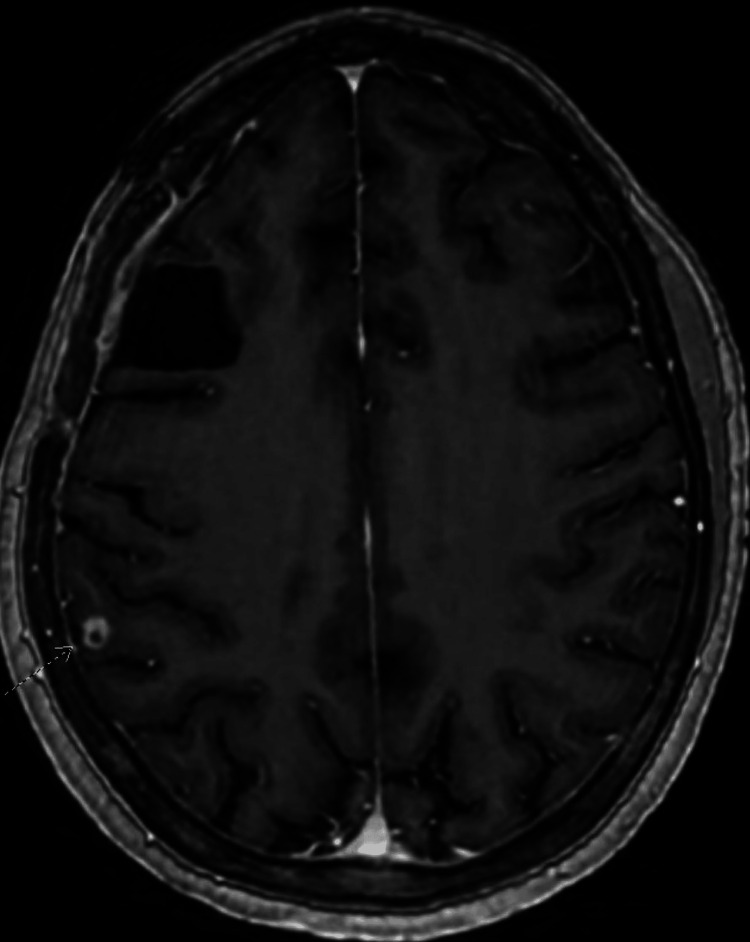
Follow-up brain MRI with and without contrast at four months after craniotomy There are resection changes in the right frontal lobe with minimal adjacent edema and numerous subcentimeter enhancing lesions with a decrease in size. These are noted by the radiologist's dotted arrow. Findings indicate a positive treatment response.
